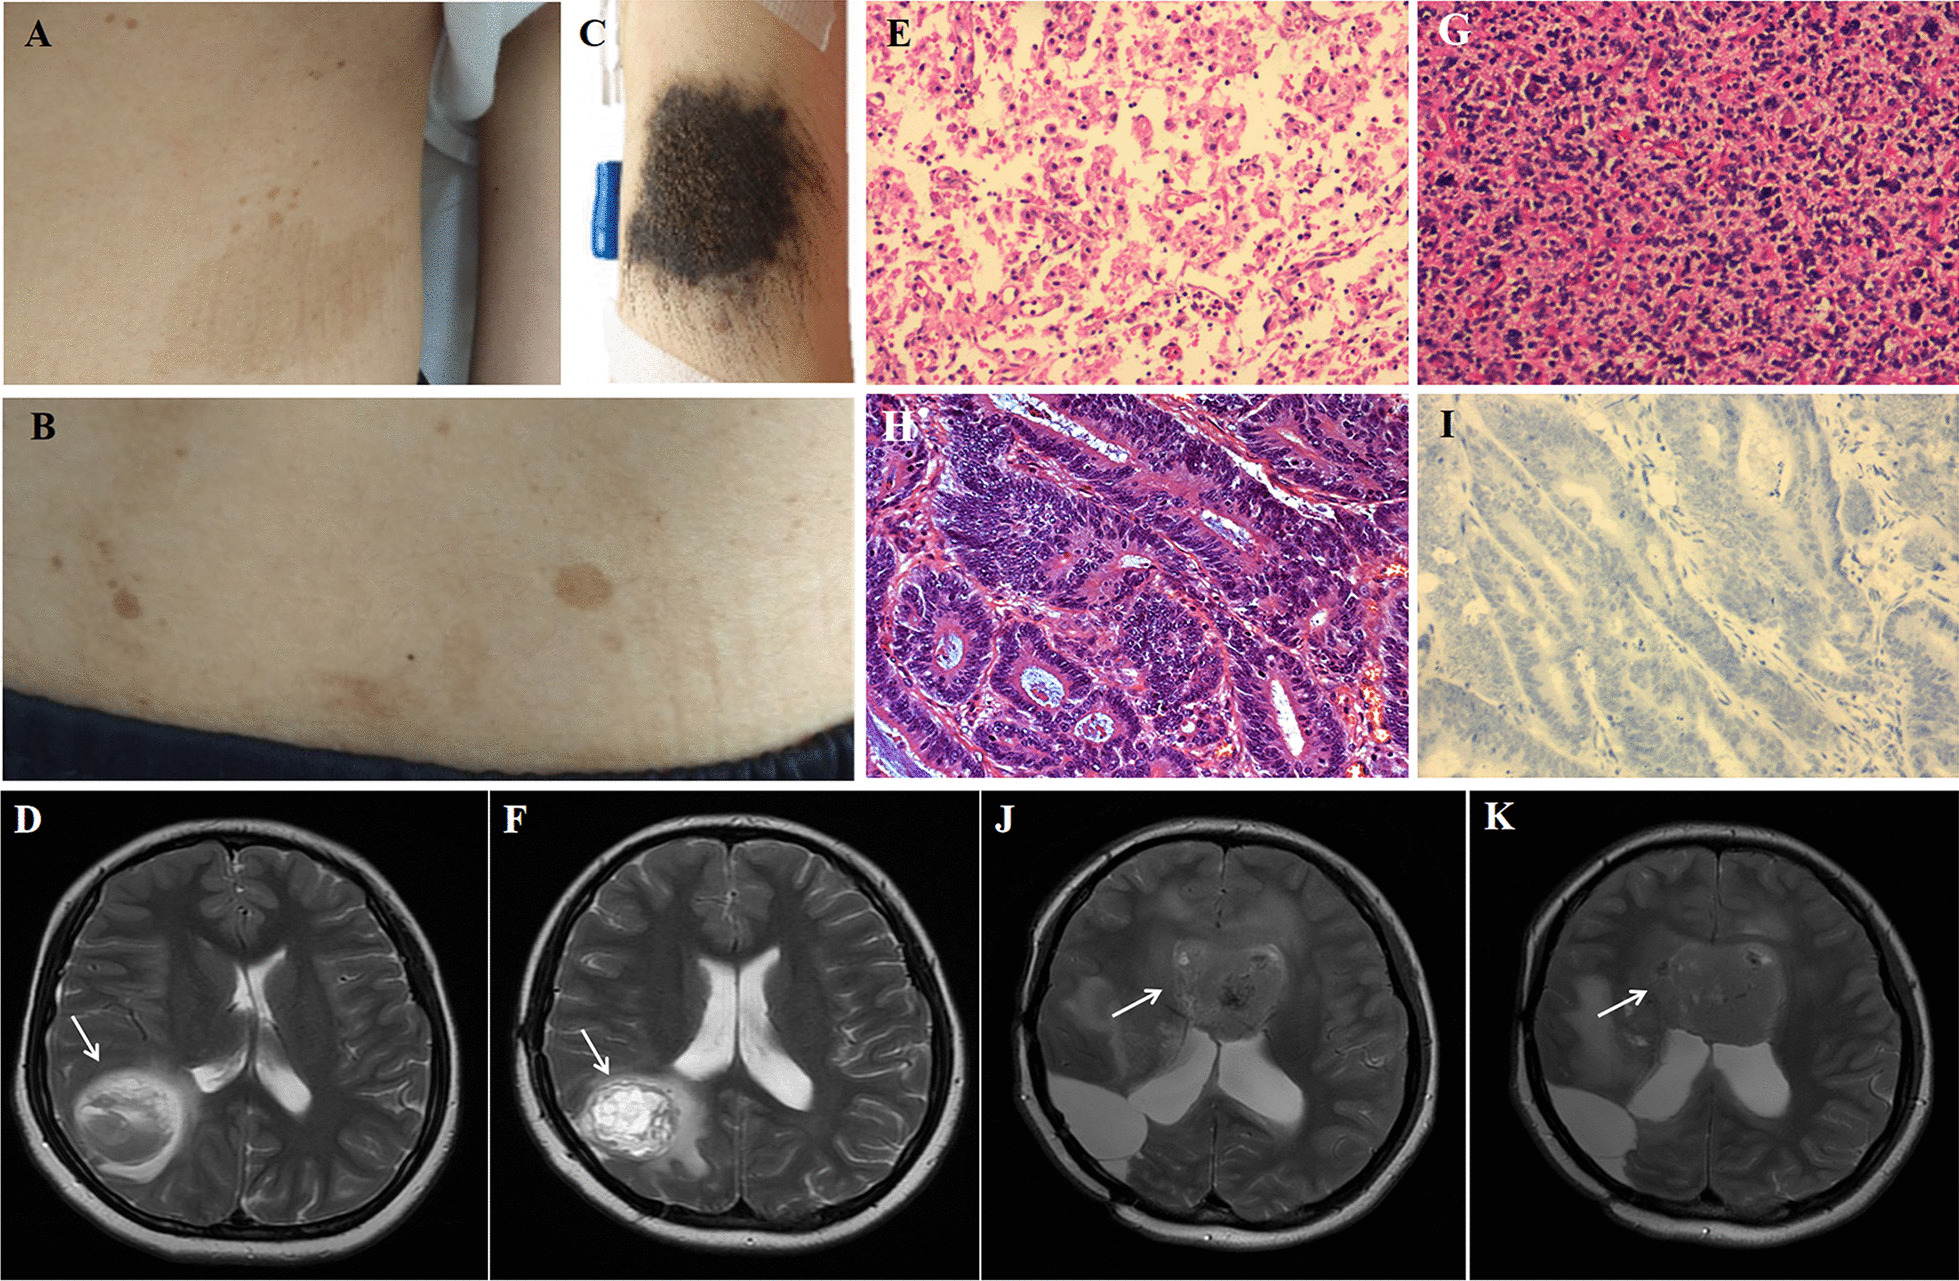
Photograph of the patient skin cafe´-au-lait macules located on (A) the anterior abdominal wall and (B) lower back and (C) a hairy pigmented cutaneous nevi located on the forearm. Pathologic findings showing (E) diffuse tumor cells, abundant focal cells with atypia, and vascular hyperplasia (HE, ×200); (G) diffuse tumor cells with different forms, multinucleated tumor cells, and focal interstitial small vessel hyperplasia (HE, ×200); (H) colon tubular adenomas, atypical tumor cells, deep-dyed big nucleolus and the mitosis were common (HE, ×200); (I) the expression loss of PMS2 proteins in both tumor and non-neoplastic tissue (IHC, ×200). MRI of the patient brain showing (D) a right temporal-occipital ring-enhancing lesion (arrow) of about 3.5 × 4.5 cm in size at the age of 16, (F) vascular enhancement of nodular mass (arrow) at the site of the previous surgery at the age of 16, (J, K) aggressive tumor relapse (arrow) at the age of 18
